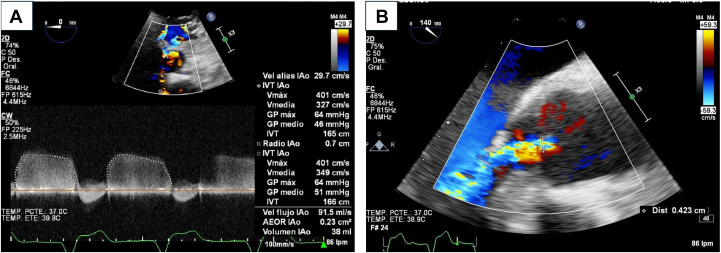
TEE and AR (A and B) Grade III/IV aortic regurgitation (effective regurgitant orifice area 0.23 cm^2^, regurgitant volume 38 mL, vena contracta 4.2 mm). AEOR IAo = effective regurgitant orifice; AR = aortic regurgitation; TEE = transesophageal echocardiogram; Volumen IAo = regurgitant volume.
